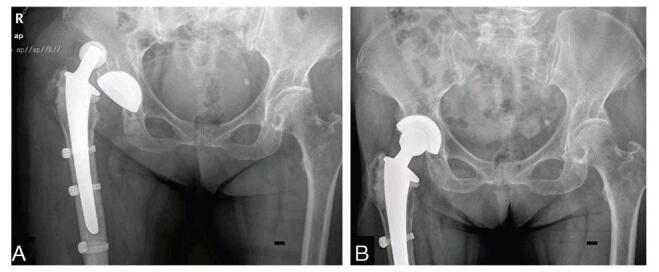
Figure 6. X-ray radiography of the hip at 4 weeks after the first revision. A, X-ray of redislocation of artificial hip. B, X-ray after the second revision. The scale bar indicates 10 mm.
